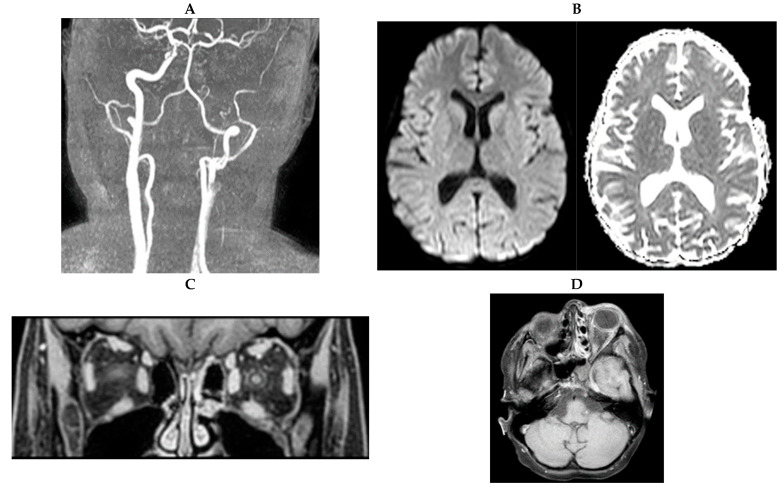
Magnetic resonance (MR) imaging on day 0. (A), time-of-flight (TOF)-angiography of the cerebral arteries reveals occlusion of the left internal carotid artery. (B), diffusion-weighted-imaging (DWI)/ apparent diffusion coefficient (ADC)-Map shows the absence of acute ischemia as explained by sufficient collateralization from the right cerebral arteries. (C), after administration of gadolinium, coronar T1w-mDIXON reveals swelling of the surrounding structures of the left optic nerve, and (D), swelling and contrast enhancement of the left intraorbital muscles.
